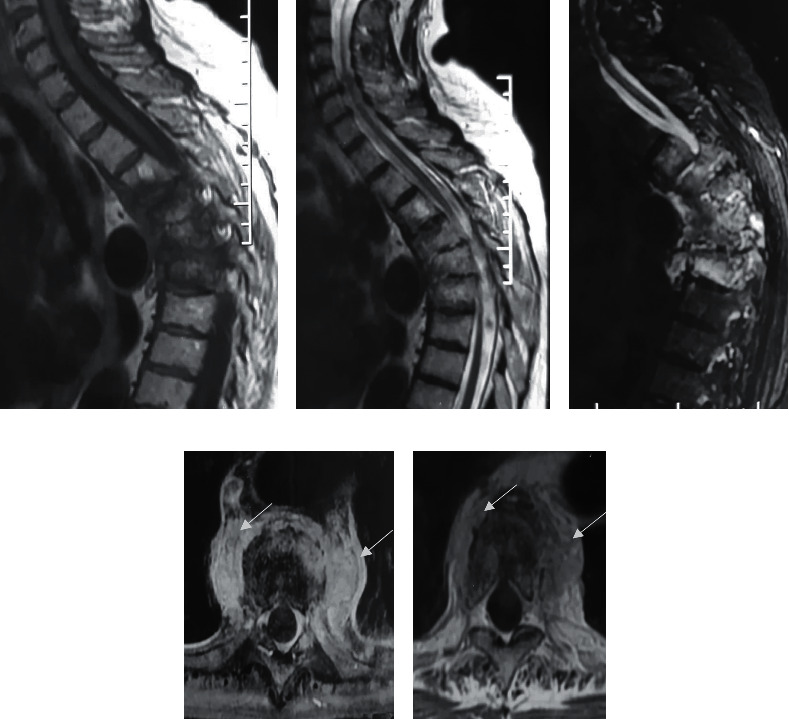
Magnetic resonance imaging. (a) Sagittal T1 WI, (b) sagittal T2 WI, and (c) sagittal STIR WI showing irregularity of the end plates from D4 to D7, high T2 signal in the disc, marrow signal change in the adjacent vertebra (T1 low and T2 high), and soft tissue mass (arrow). (d, e) Magnetic resonance imaging axial T1 after contrast showing soft tissue microabscess (arrow).
